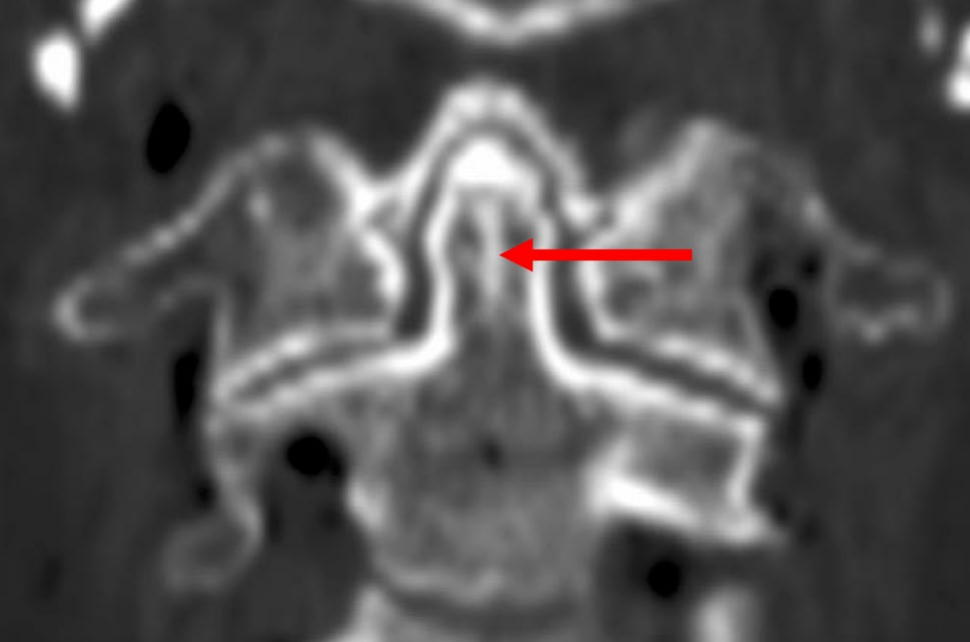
Coronal computed tomographic image. A highly ossified region in the midline of the odontoid process which looks like a bony septum (arrow)
